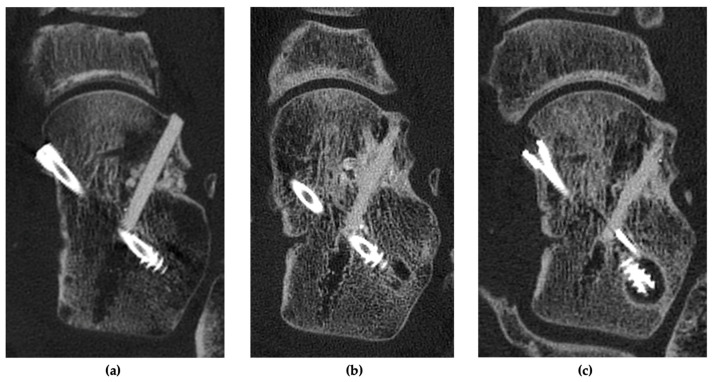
Axial CT scans of the reconstructed talar neck fracture with medially placed compression screws and laterally placed allograft bone screw with bone replacement material, showing the follow-up after three months (a), one year (b) and two years (c) with the osseointegration of the intact allograft bone screw without lateral column shortening (valgus collapse).
